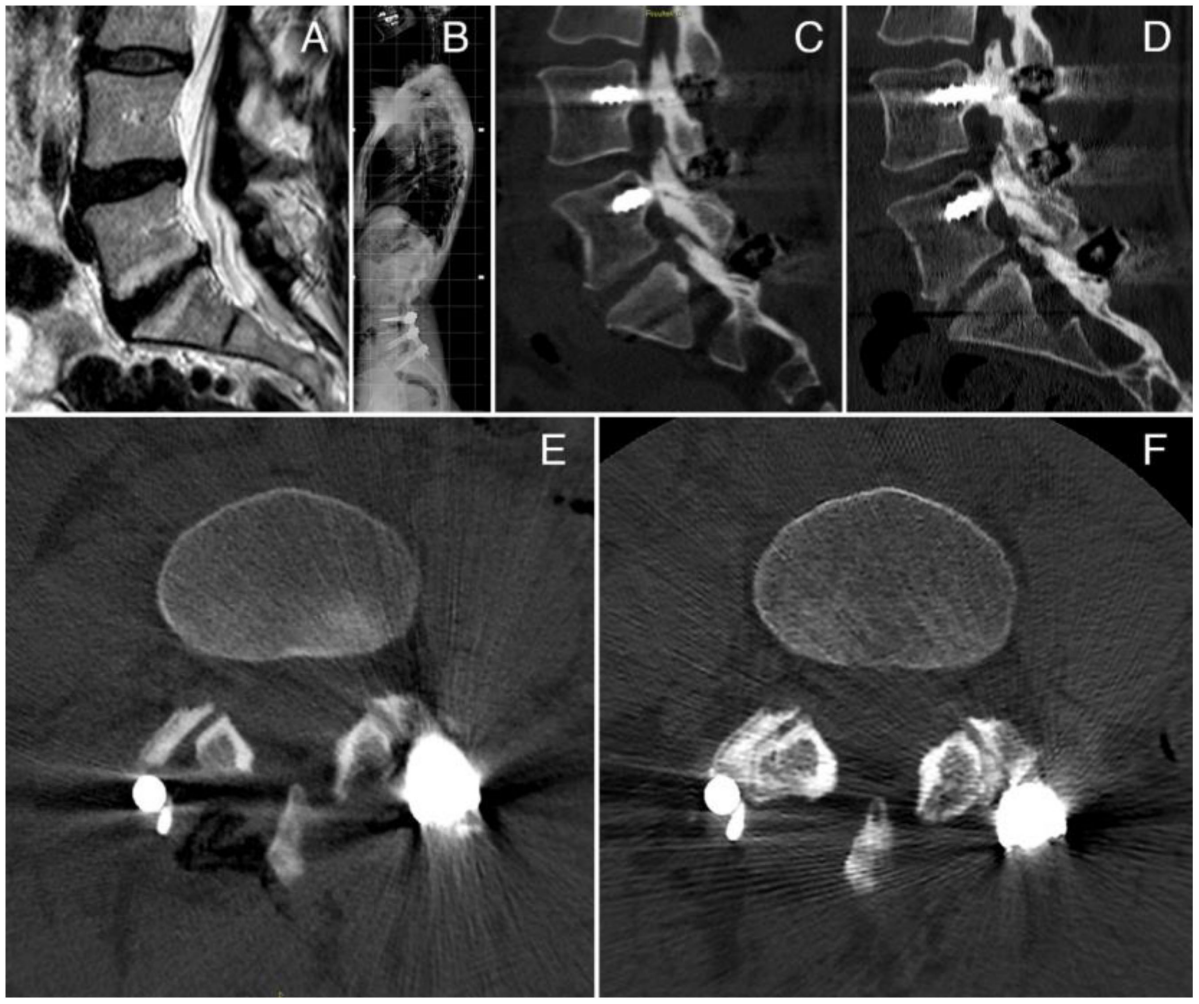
(A) Preoperative MRI; (B) postoperative X-ray; (C) CT scan sagittal view at 6 months of follow-up; (D) CT scan sagittal view at 12 months of follow-up, showing a large amount of bone formation at the L4–L5 and L5–S1 levels; (E) CT scan axial view at 6 months of follow up; (F) CT scan axial view at 12 months of follow-up, showing bone formation at the L4–L5 facet joint level.
